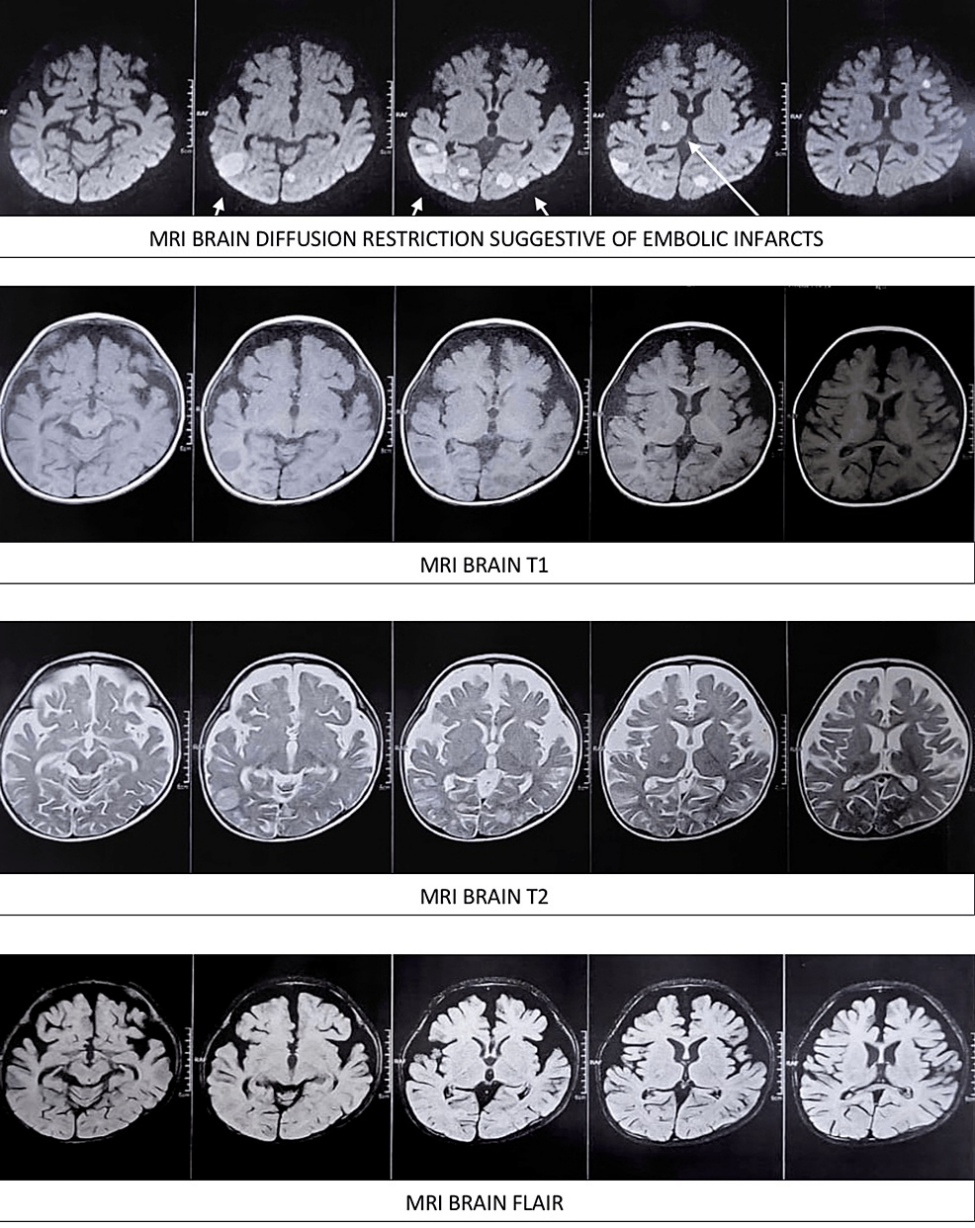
Magnetic resonance imaging (MRI) brain (diffusion restriction, T1, T2, Flair) (top to bottom) revealed areas of diffusion-restricted embolic infarcts with diffuse leptomeningeal enhancement and mild cerebral edema
